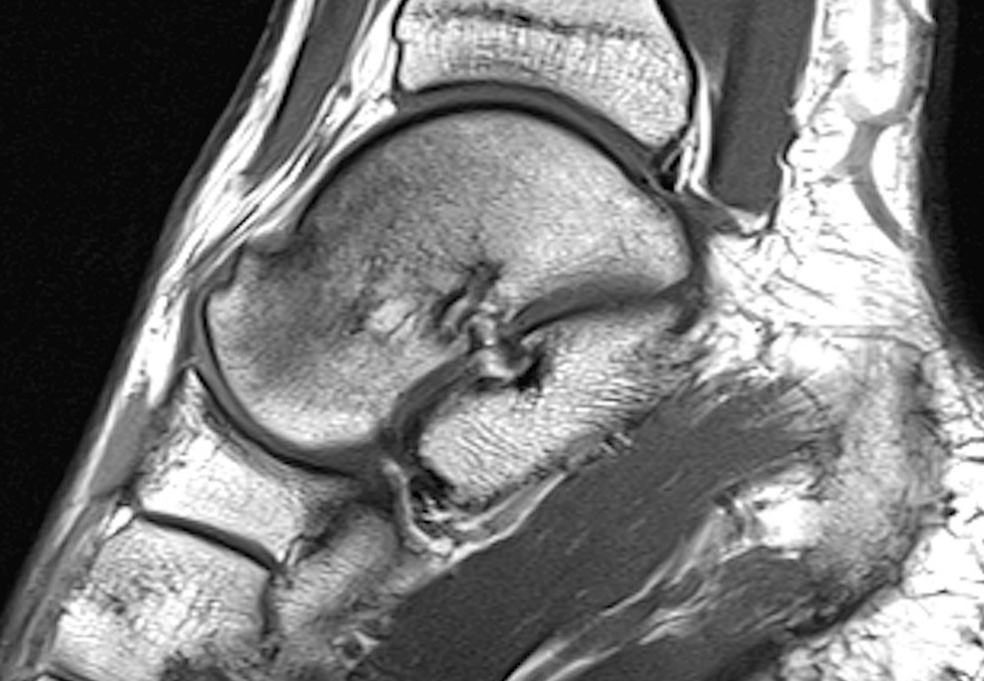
A second MRI scan, two months after his symptoms presented, showed no improvement compared to the initial findings.
